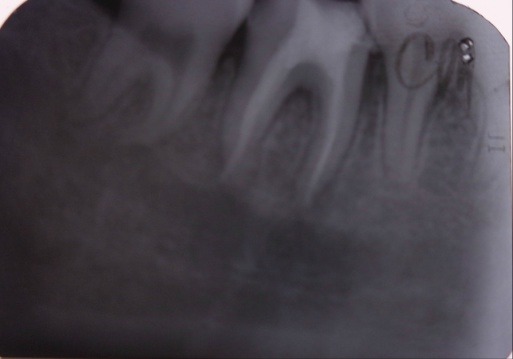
Tooth 4.7 with a decreased periapical and furcation radiolucency
